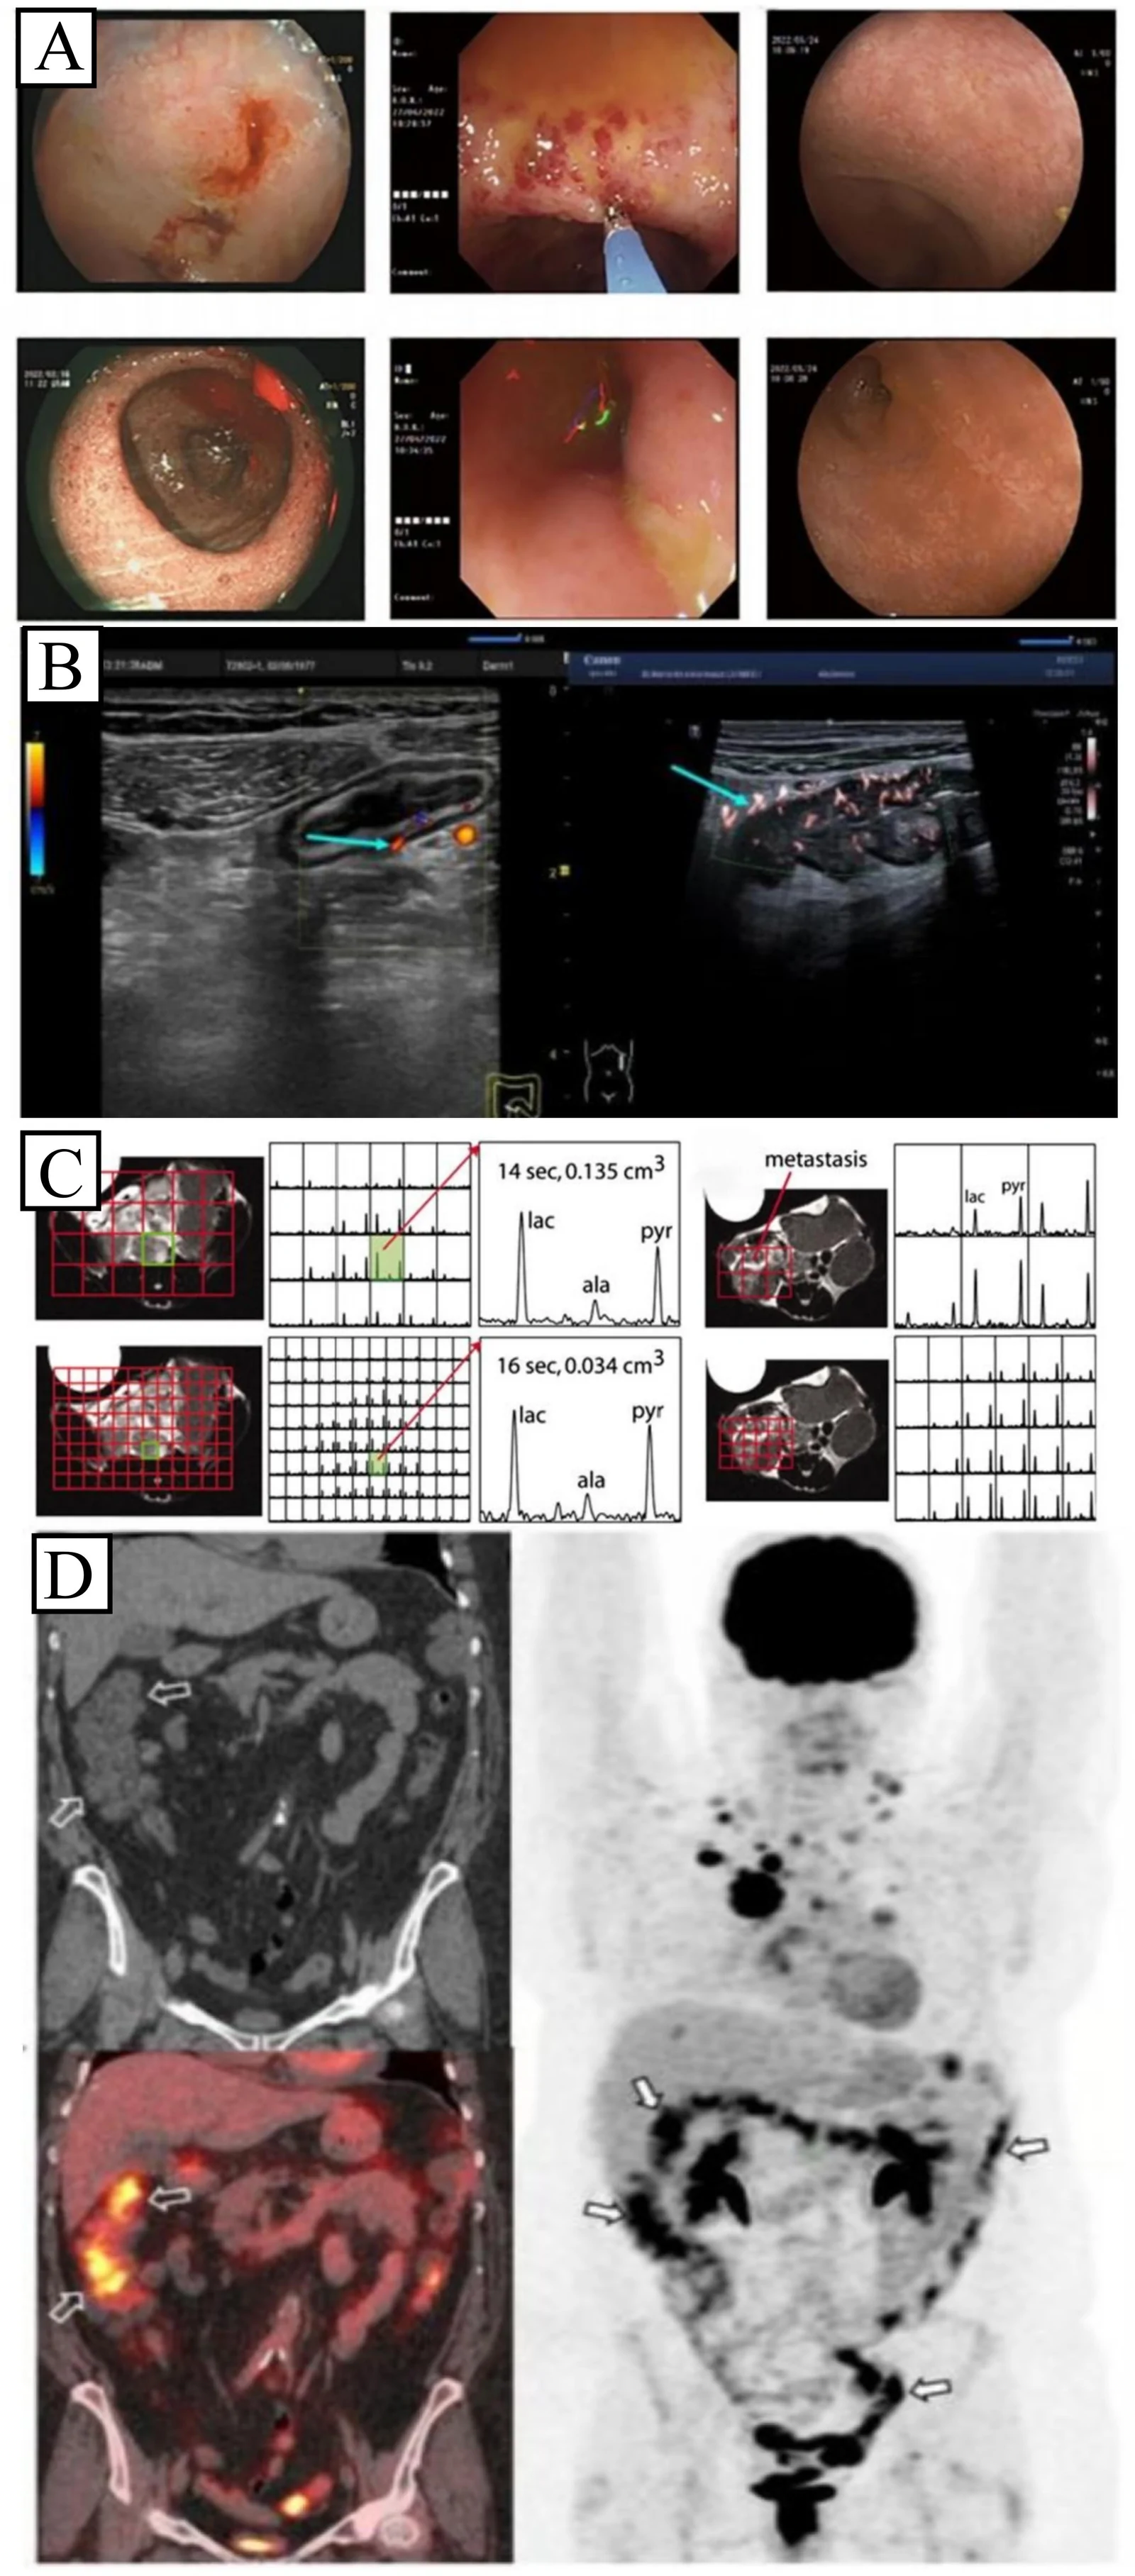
Application of multiple imaging techniques for myokine-associated immune-related colorectal injury. (A) Serial NBI colonoscopy presents mucosal hyperemia, erosion and ulceration in PD-1 inhibitor-induced colitis combined with Cytomegalovirus infection (83). (B) Color doppler EUS shows thickened intestinal wall and hypervascular signals indicating transmural inflammation and early fibrosis (84). (C) Accelerated MRSI visualizes pyruvate, alanine and lactate metabolic distribution. (D) FDG positron emission tomography-computed tomography fusion locates hypermetabolic segments with massive immune cell infiltration (85).
